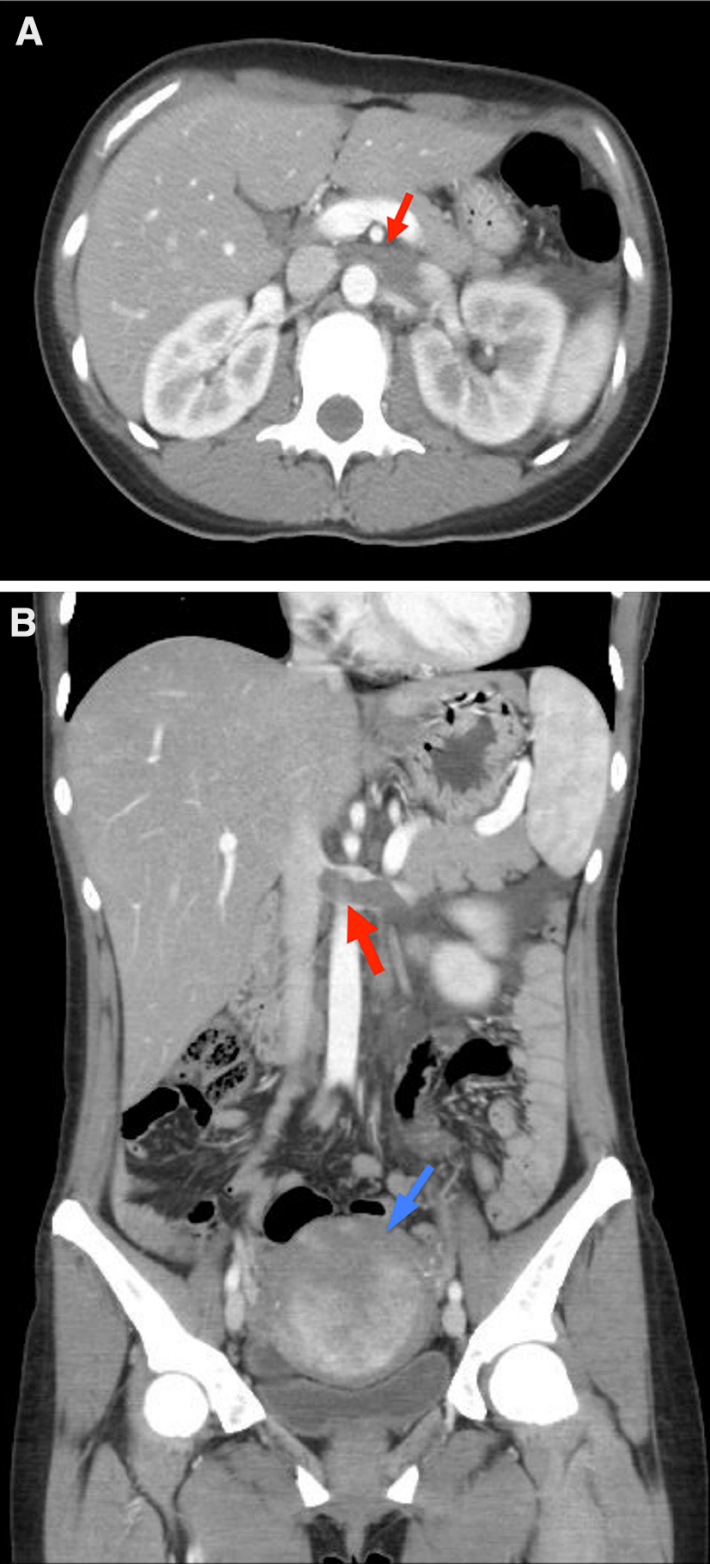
a An axial image of abodominal CT scan with contrast material. A large thrombus occluded the left renal vein (*red arrow*). b A coronal image of abdominal CT scan with contrast material. A large thrombus occluded the left renal vein (*red arrow*). The uterus was enlarged due to adenomyosis uteri and myoma uteri (*blue arrow*)
